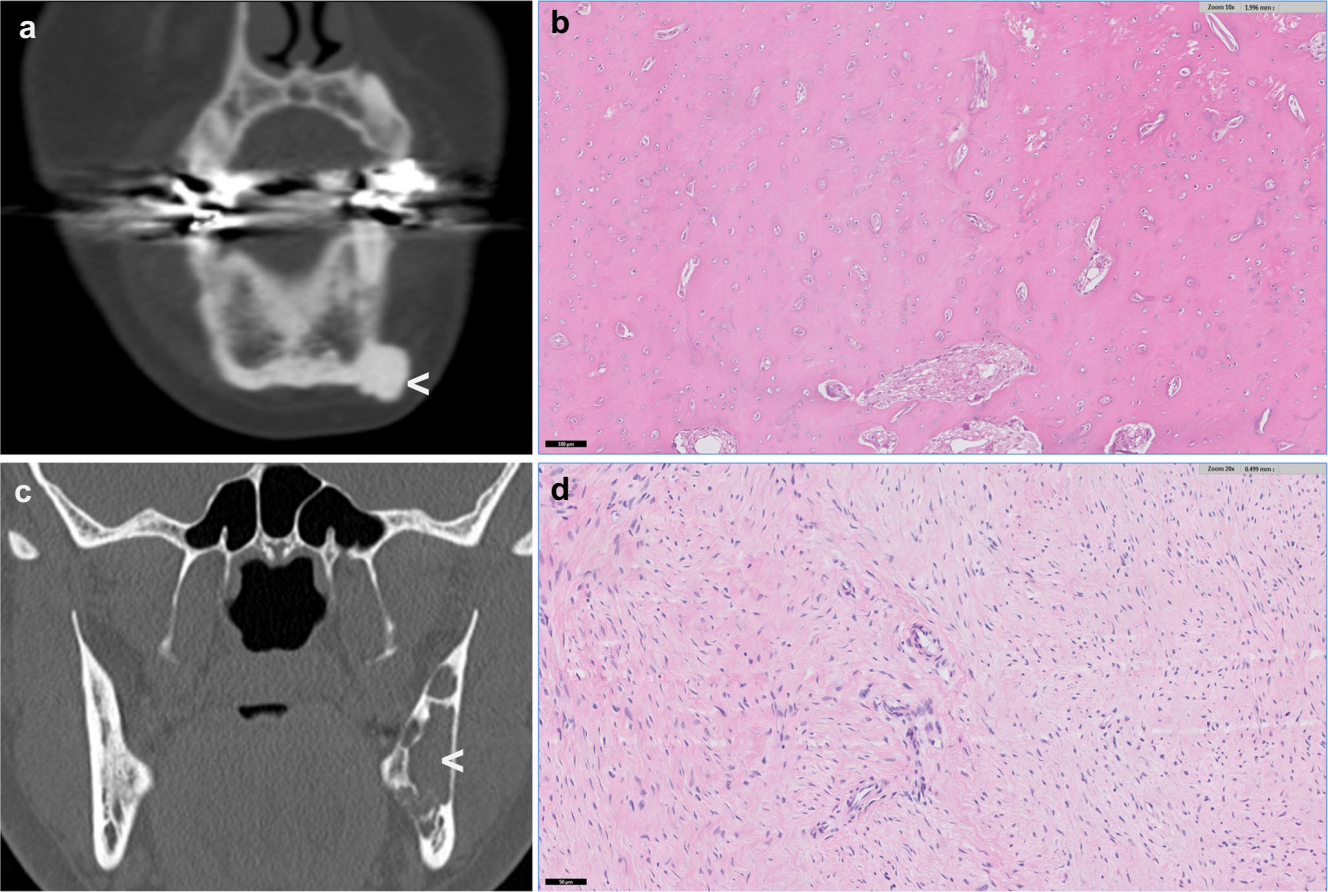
Radiology and morphology examples of benign maxillofacial bone tumours. a Coronal reformatted CT image demonstrating the homogenous sclerotic mass of an osteoma arising from the inferior surface of the mandible (arrowhead). b HE slide showing osteoma composed of compact mature lamellar bone. c Coronal reformatted CT image of a desmoplastic fibroma showing a well-defined osteolytic lesion in the angulus of the mandible on the left side (arrowhead). Cortical erosion and internal septations but no soft-tissue extension. d Morphology of a desmoplastic fibroma showing a cellular proliferation of spindle to stellate cells arranged in long fascicles in a collagen-rich matrix
